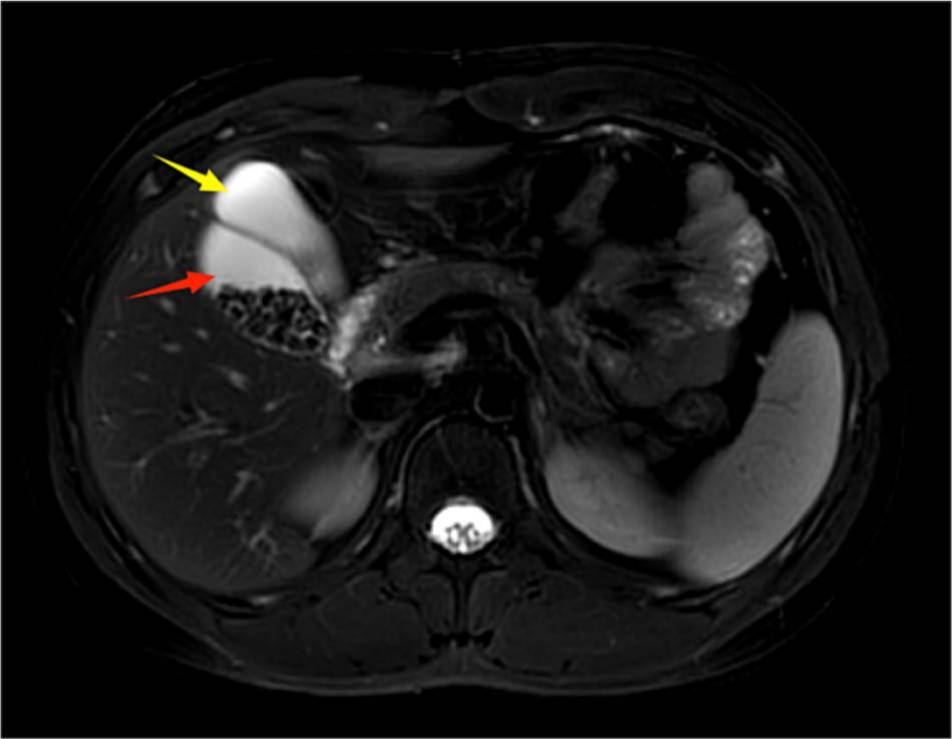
Upper abdominal MRI demonstrates a double gallbladder variation. The two arrows indicate the respective gallbladders, the lateral one of which contains multiple gallstones.
